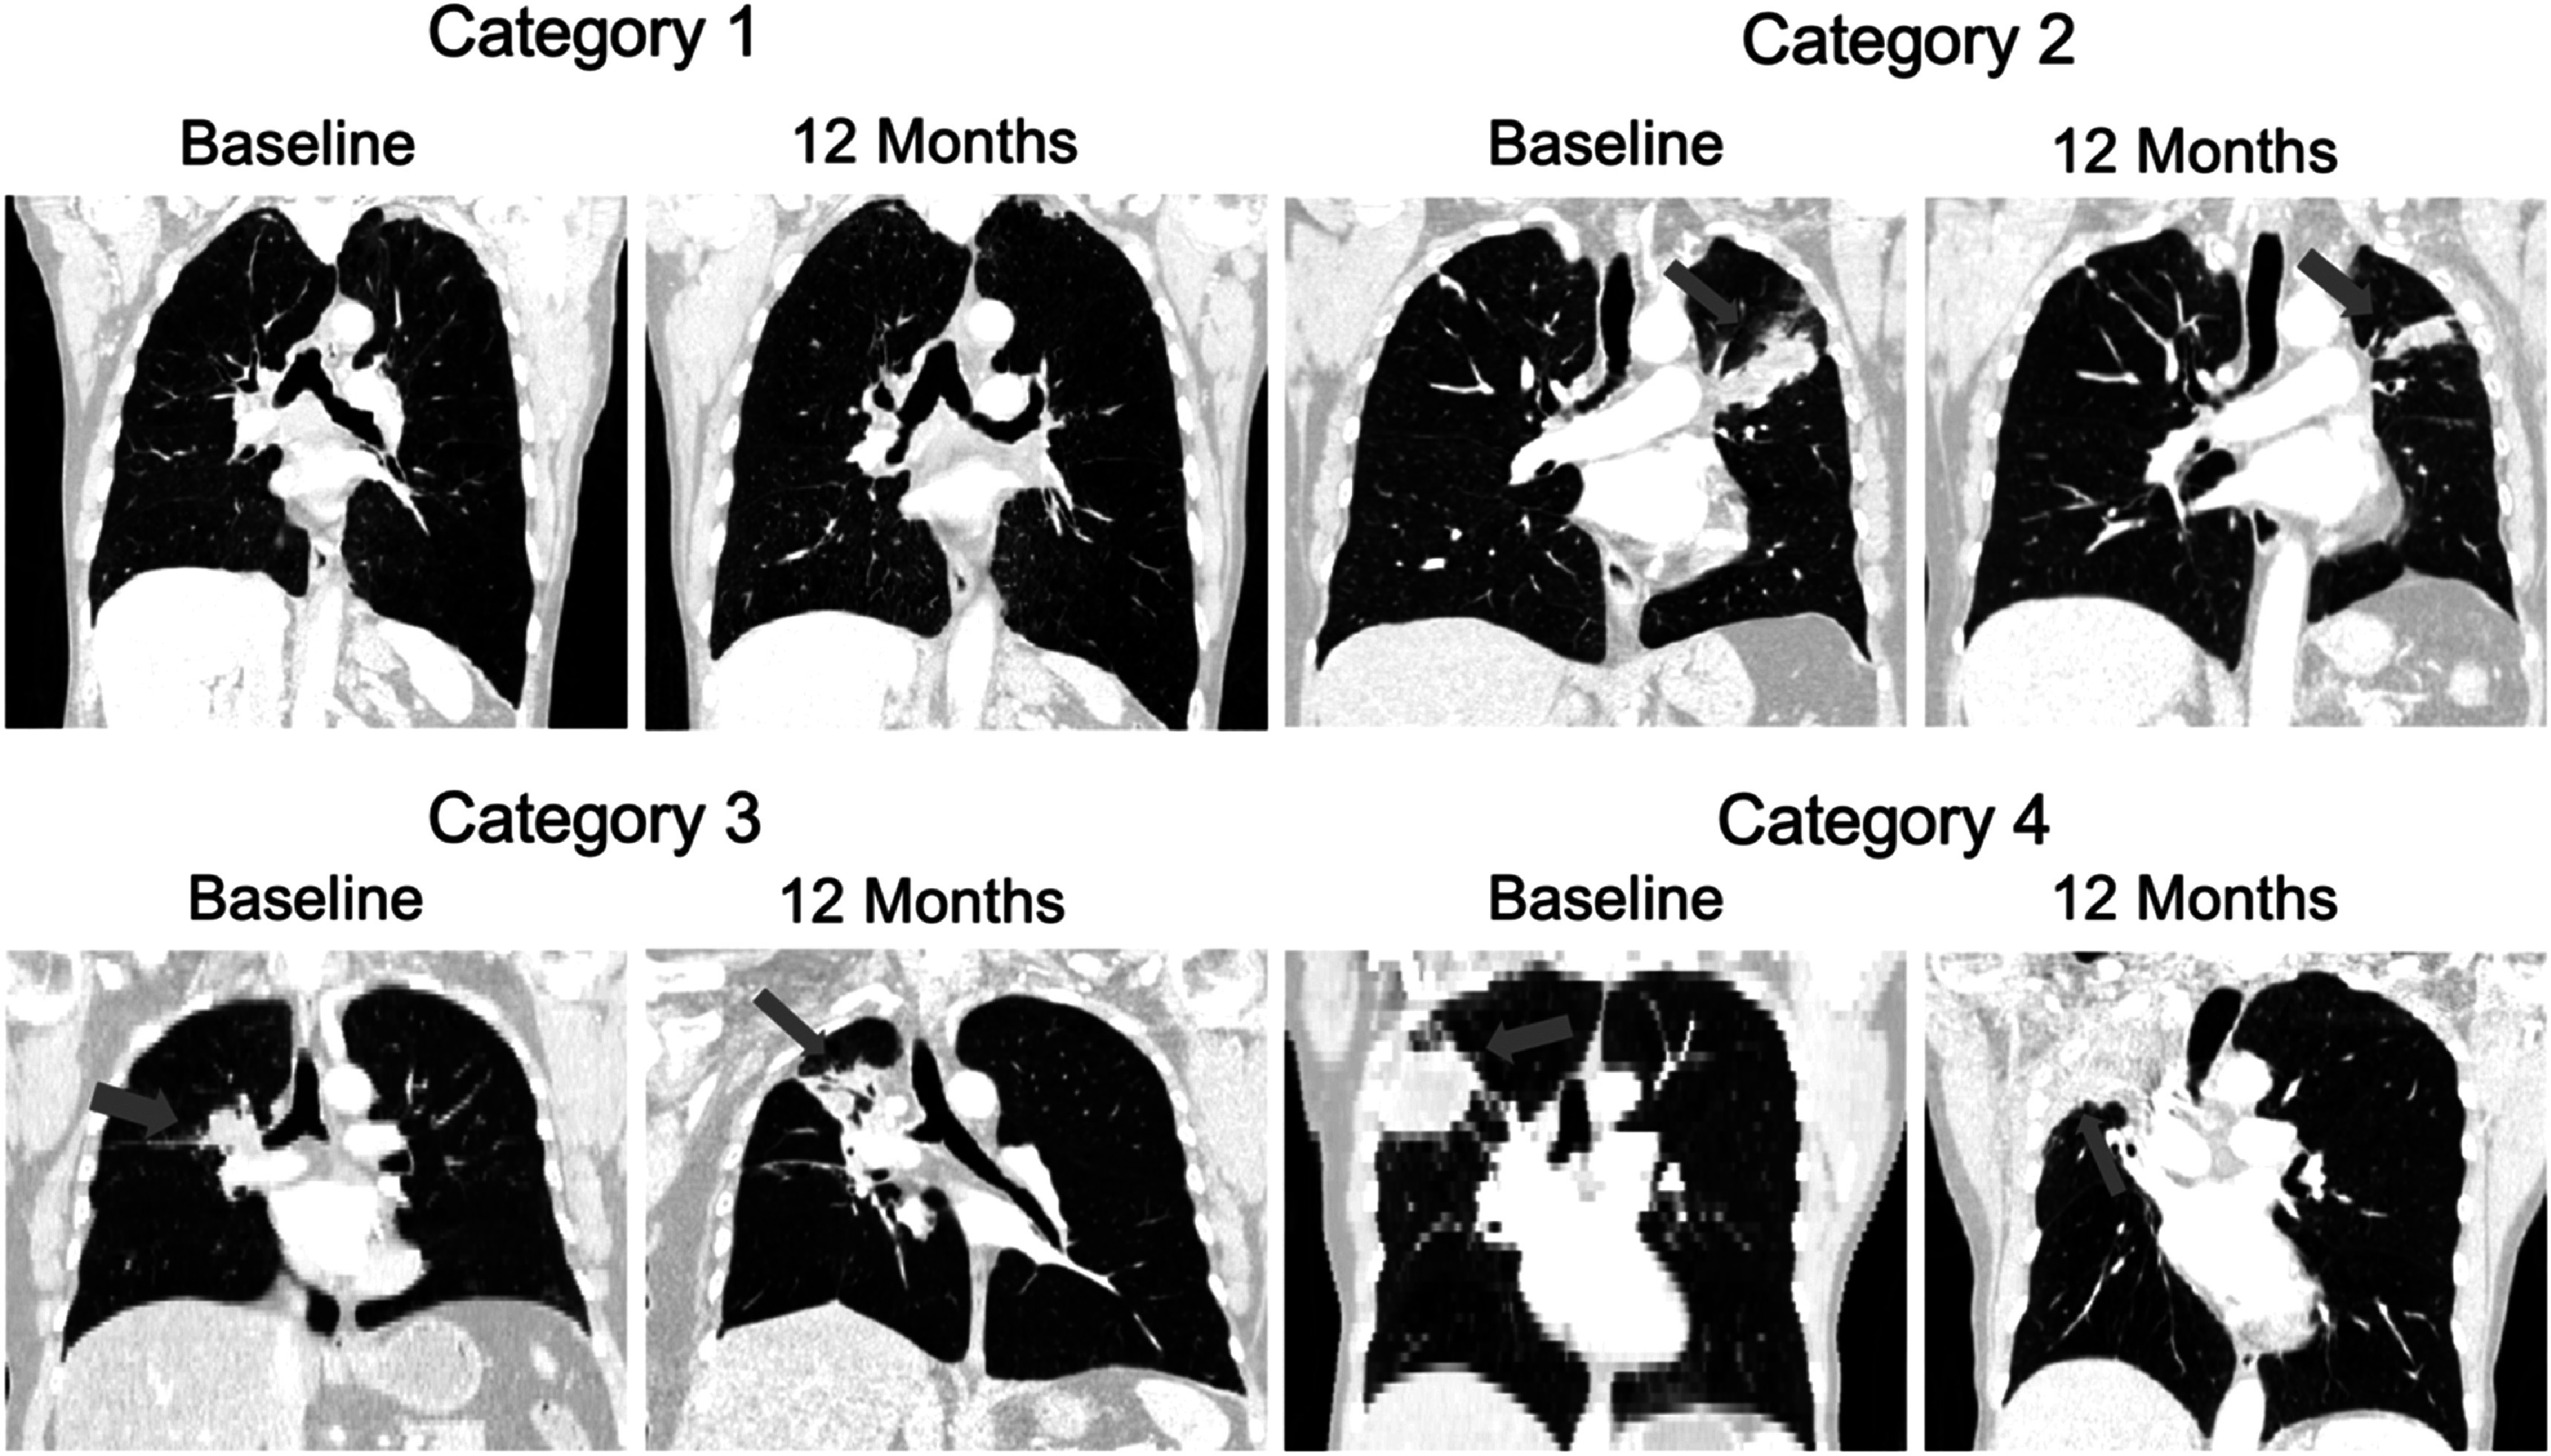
Baseline and 12 month follow up CT scan pairs of four patients with increasing registration difficulty. It can be seen that the scans in category 1 are very similar to each other, with most differences resulting from tumour regression in the left lung and inhalation level of the scans. In category 2, there are noticeable parenchymal and lung volume differences in the ipsilateral lung (left), but mainly contained in the upper lobe where the tumour is located. In category 3 such differences between the scans become more pronounced. Consolidated tissue is present in all three ipsilateral (right) lobes of the 12 month scan and the damage has extended past the region the tumour originally occupied. There are also changes in the size and shape of the ipsilateral lung between timepoints, with diaphragm tenting present in the follow up scan. In category 4, the most extreme changes are present with the upper right lobe completely collapsed in post-RT. The resolution is very different between the baseline (5 mm slice thickness) and follow up (1 mm slice thickness) scans, adding to the challenge in the registration.
